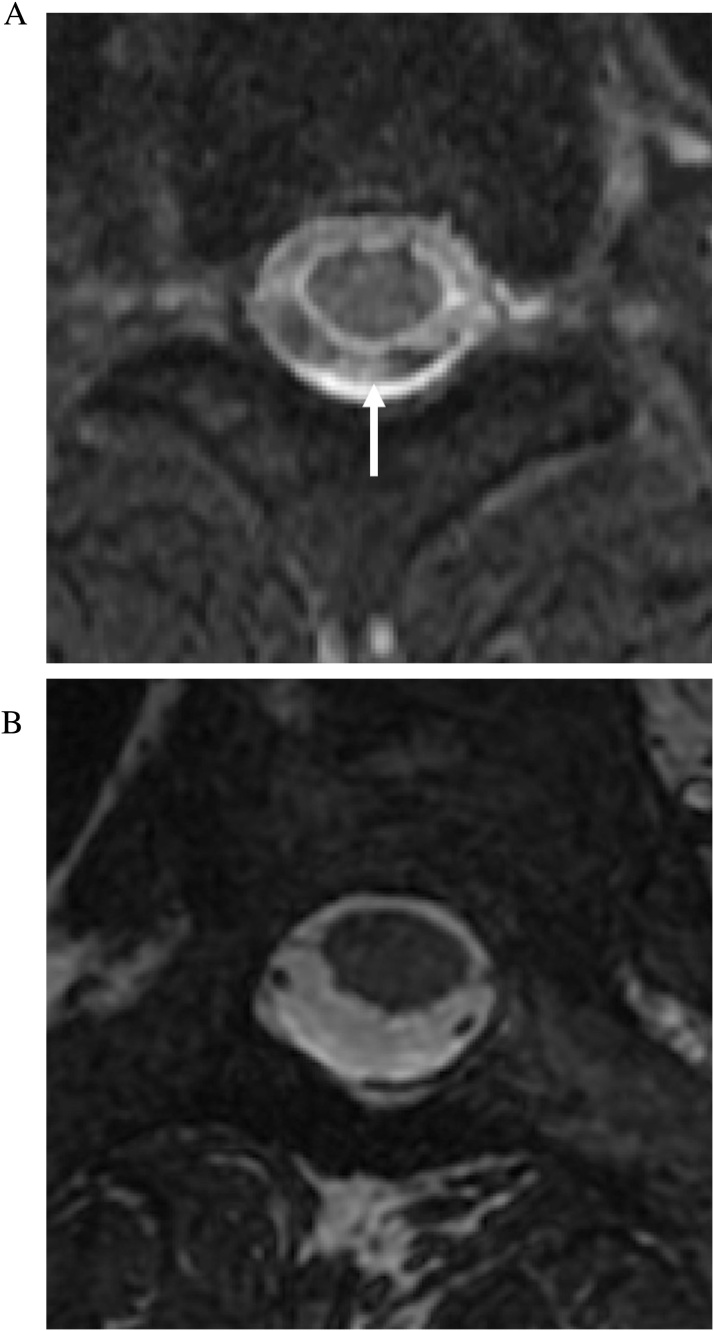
CSF pulsation artifact. (a) Axial T2-weighted FSE MRI image (slice thickness, 3 mm) of the upper cervical spine demonstrates CSF pulsation artifact within the thecal sac, more prominent at the dorsal aspect (arrow). (b) Axial FIESTA-C MRI image (slice thickness, 1.2 mm) through the same level demonstrates homogeneous high signal of CSF and elimination of the pulsation artifact.
